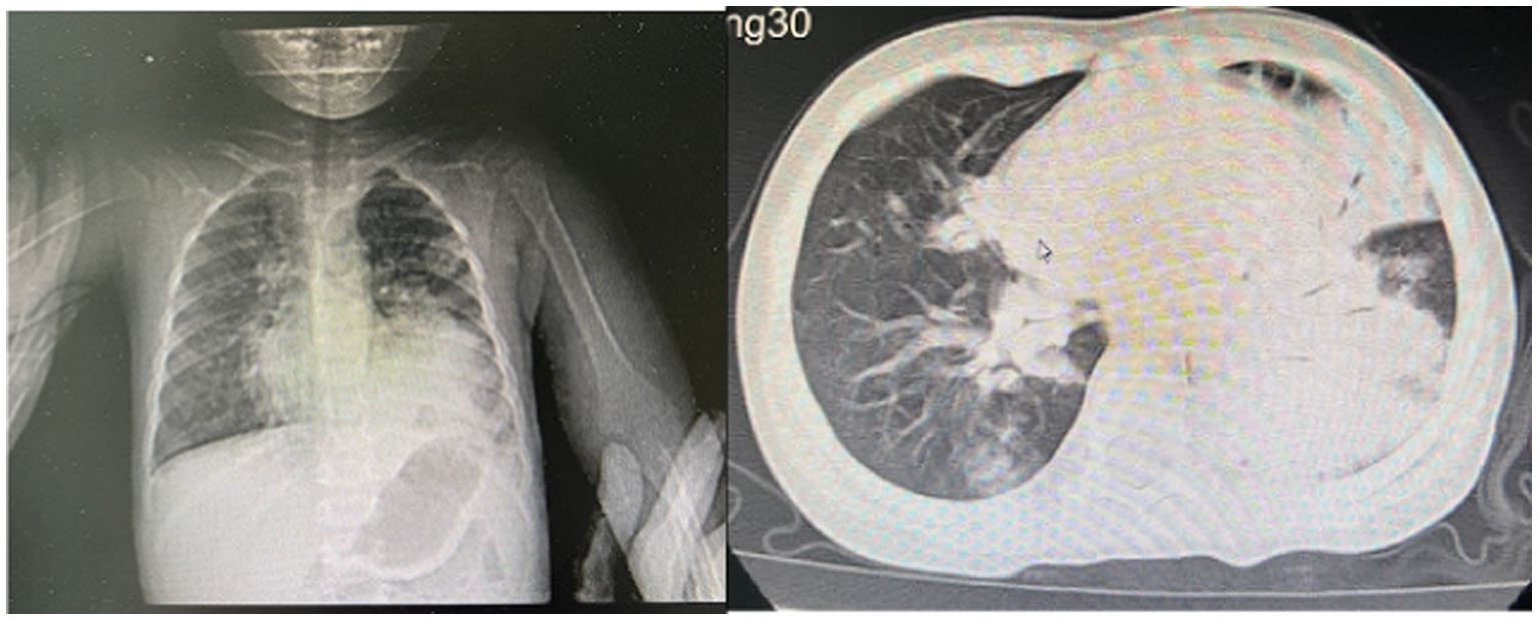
Chest CT scan on the 6th day of the illness.
